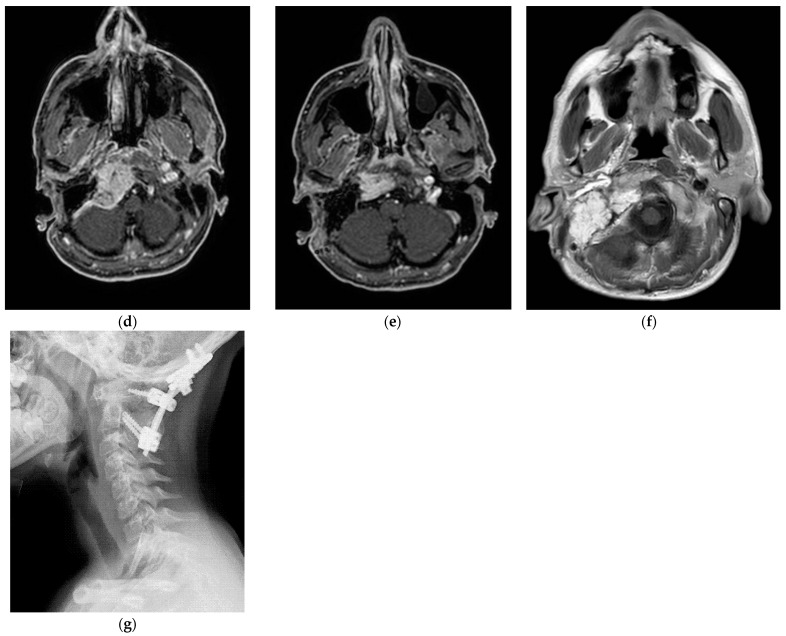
(Case 5) (a,b) Right tympanojugular paraganglioma (C3Di2Vi) involving the clivus, the vertebral artery, the foramen magnum, and the occipital condyle. (c) Axial-enhanced T1-weighted magnetic resonance imaging (MRI) shows a large mass extending to the intradural space up to the foramen magnum. (d) Axial-enhanced T1 MRI after first-stage tumor removal. (e) Enhanced T1 MRI after second-stage surgery. (d,f) Axial-enhanced T1 MRI after third-stage tumor removal, which shows total tumor removal with obliteration of the surgical cavity with abdominal fat. (g) A sagittal CT scan after total tumor removal during the third stage shows cervical–occipital fixation.
